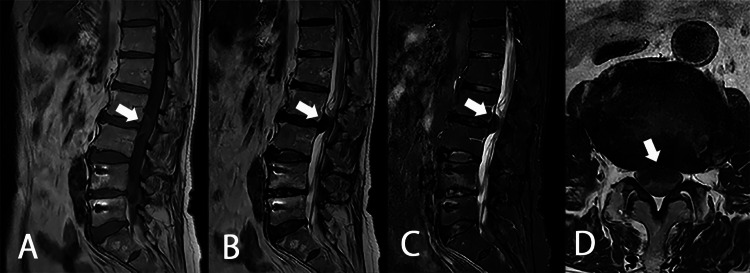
MRI scans of case 2, three years after the first TLIF operation A: T1WI sagittal section; B: T2WI; C: STIR; D: T2WI axial section. Extramedullary mass located in L2/3 intervertebral disc level (white arrows). The initial radiological diagnosis was a herniated disc MRI: magnetic resonance imaging; TLIF: transforaminal lumbar interbody fusion; STIR: short tau inversion recovery
